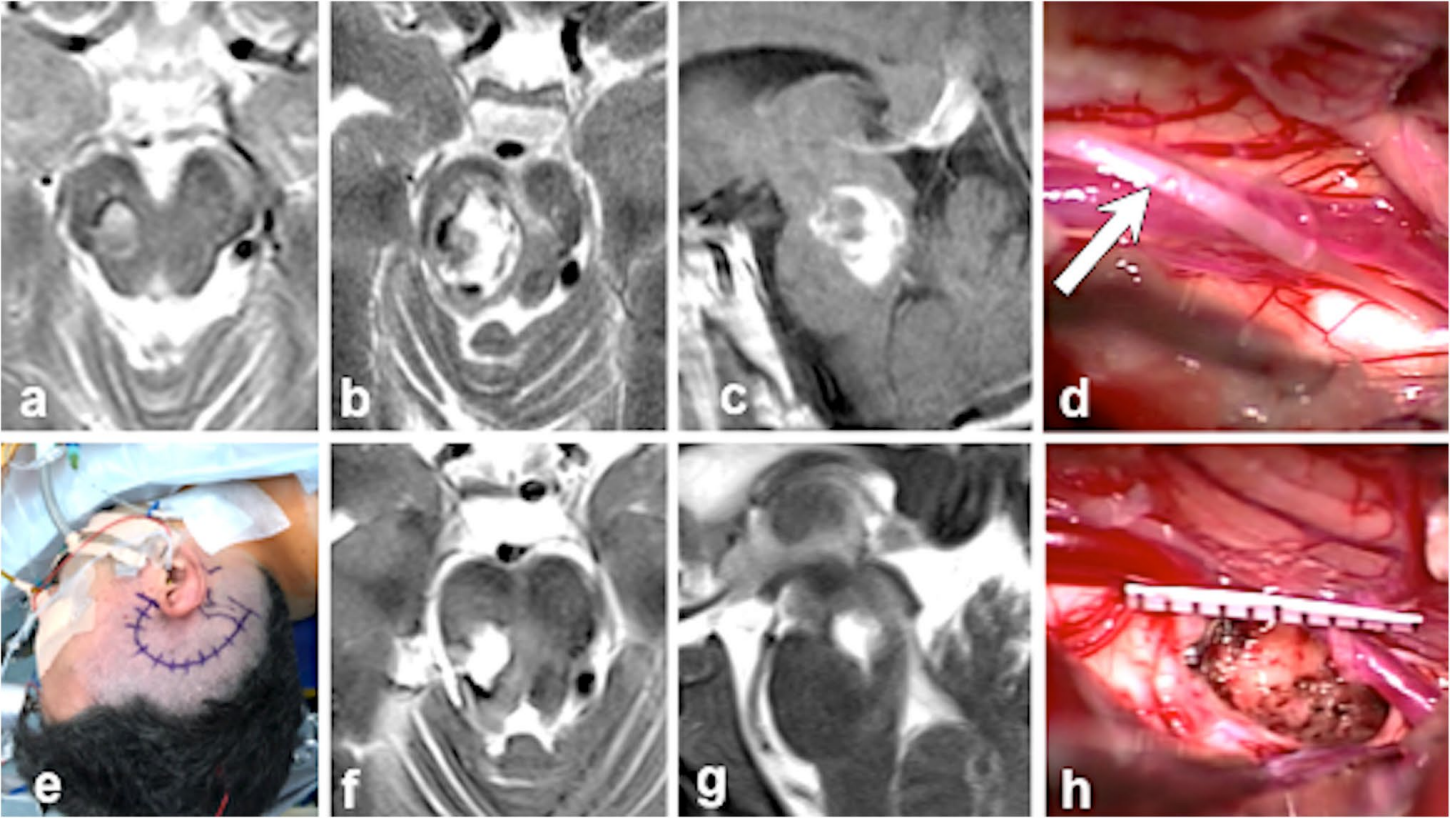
This 36-year-old male harbored multiple cavernous malformations in the basal ganglia, cerebellum, and midbrain. Three years prior to surgery, he suffered the first brainstem hemorrhage, fortunately without neurological deficit. Six months before surgery, a slight hemorrhage was noted on MRI (a), but the patient was asymptomatic by that time; 3 weeks before admission, a new bleeding episode occurred that caused left-sided hemiparesis and hemihypesthesia, right sixth and seventh nerve palsy, and deteriorated consciousness. Preoperative axial (b) and sagittal MRI showed a 22-mm hemorrhagic MCM extending caudally (c). He underwent surgery via the right subtemporal approach; intraoperatively, the surface of the tegmentum was prominent at the level of the fourth cranial nerve (*arrow*, d). Surgery was performed with the patient in the supine position (e). Postoperative axial (f) and sagittal (g) MRI as well as the intraoperative photograph (h) confirmed complete MCM removal. The patient rapidly recovered after surgery, and after 6 months, he remained without permanent neurological deficits
